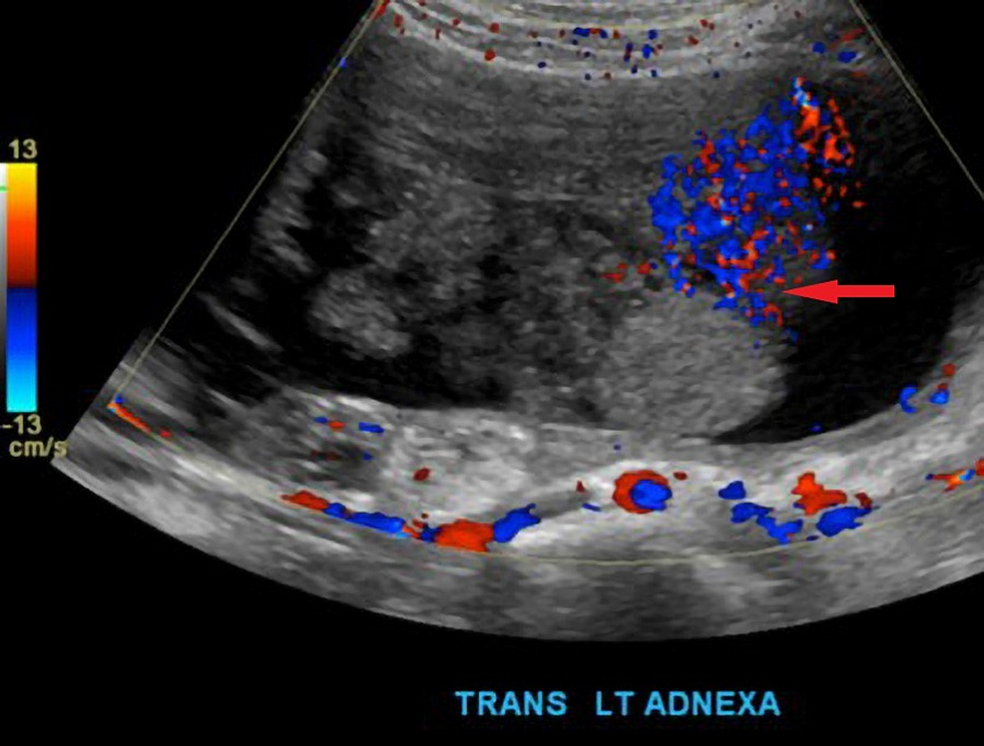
Magnification grey and color doppler images of the left adnexa show a complex mass with a cystic and solid component, dermoid plug (red arrow).
